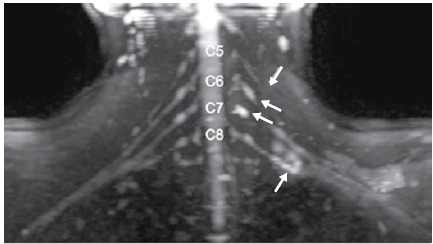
Magnetic resonance imaging, patient 3. Diffusion-weighted neurography - reconstruction in the coronal plane: Thickening and increased signal in the C5 root and region of the division of the lower trunk. Formation of pseudo-meningocele in the emergence of the C6 and C7 roots, suggestive of avulsion (pre-ganglionic lesion).
